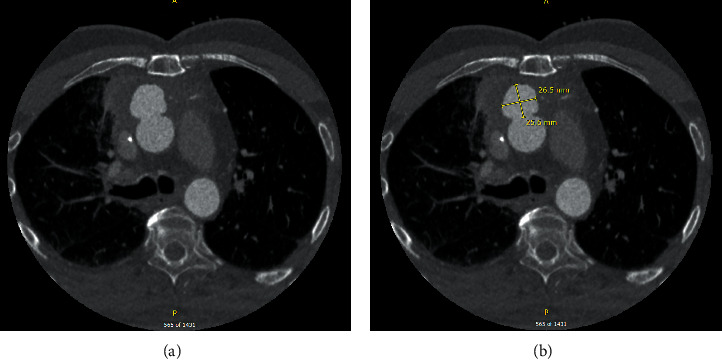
Computed tomography angiography demonstrating a large aortic pseudoaneurysm within the anterior ascending aorta.
